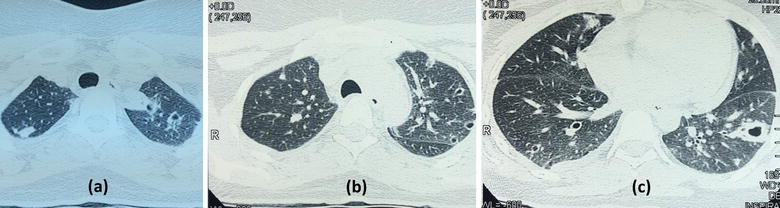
HRCT of Upper (a), Middle (b), Lower (c) Zones of the lungs showing multiple bilateral cavitary lesions without air-fluid levels
